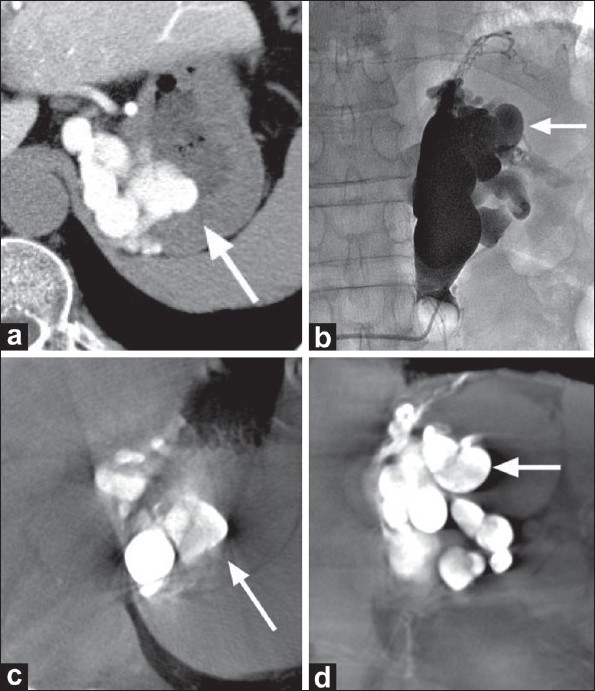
Comparative images during balloon-occluded retrograde transvenous sclerotherapy for gastric varices in a 64-yearold man with liver cirrhosis related to hepatitis C. Axial CT scans during arterial portography (a) and contrast-enhanced C-arm CT (c) both show gastric varices located at the gastric fornix with the same configuration (arrows). Frontal image of a balloon-occluded retrograde venogram from the left adrenal vein (b) delineates probable gastric varices (arrow) that were confirmed as target varices by referring to the corresponding contrast-enhanced C-arm CT coronal image (arrow) (d)
